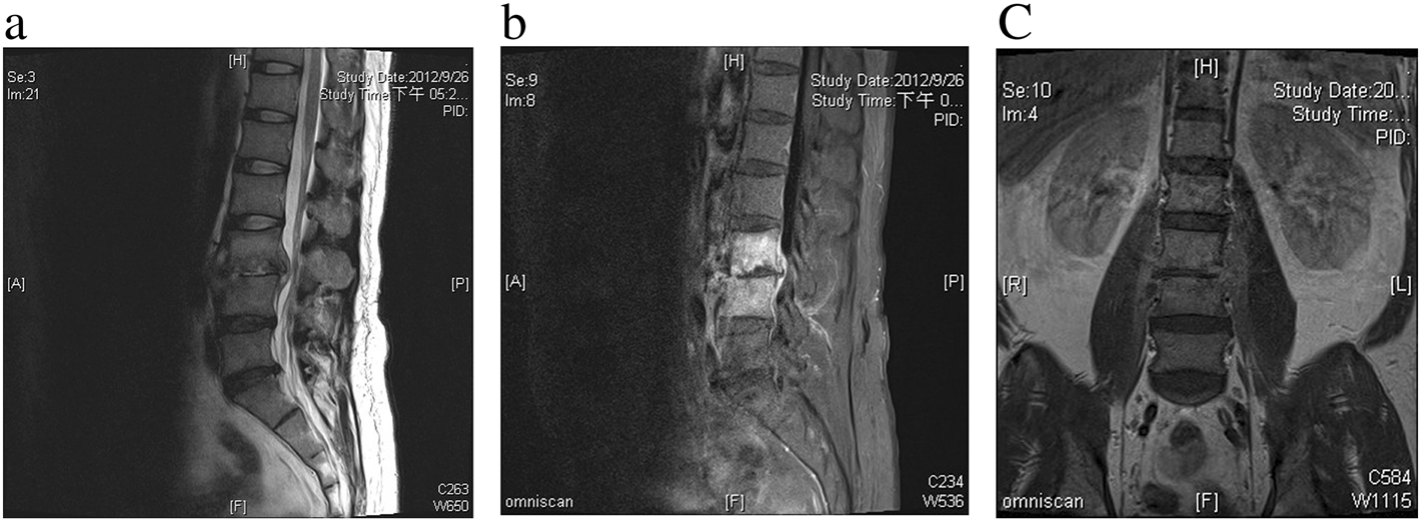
Magnetic resonance imaging of the lumbar spine showed diffuse bone marrow infiltration plus endplate erosion level at L3 and L4. (a, b, and c) Magnetic resonance imaging (MRI) of the lumbar spine showed diffuse bone marrow infiltration plus endplate erosion level at L3 and L4 characterized by low signal intensity on the T1-weighted image and high signal intensity on the T2-weighted image, with enhancement in affected bodies and cystic enhanced lesions in epidural and paraspinal regions after administration of gadolinium.
